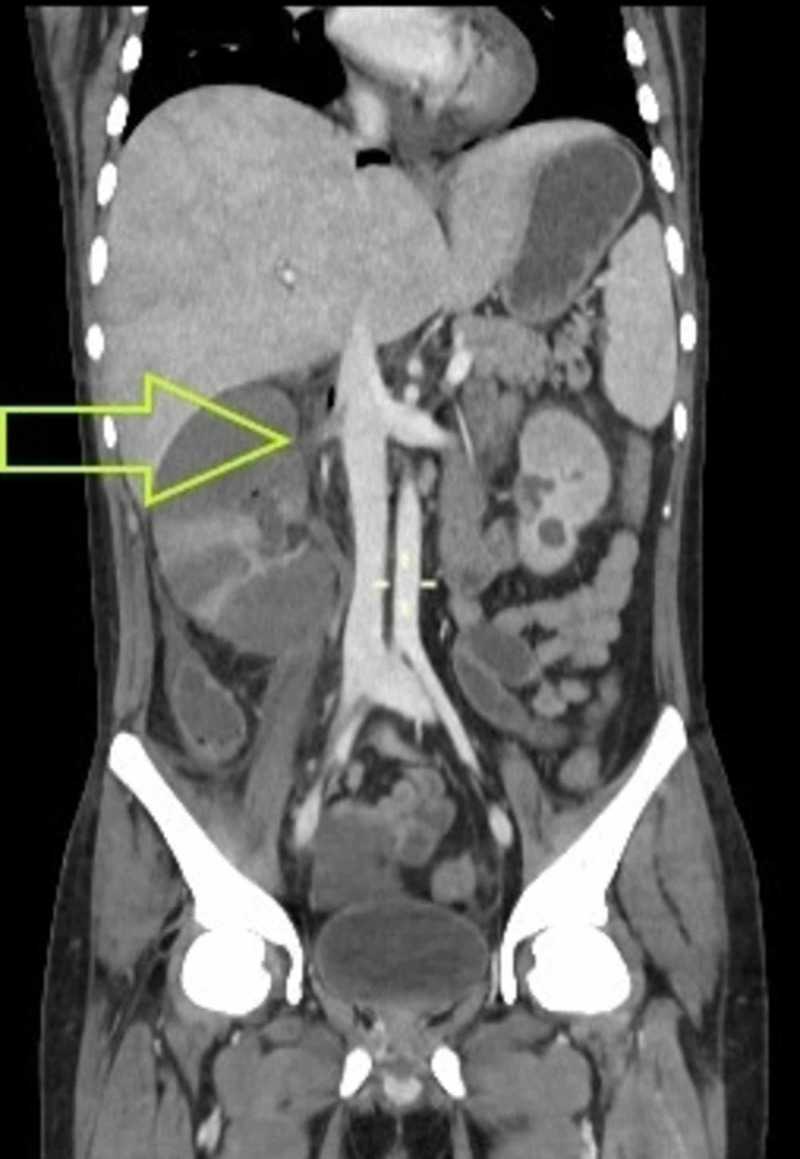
CT with contrast, axial section showing right renal vein thrombosis extending into the inferior vena cava Filling defect is noted involving the right renal vein at it ostium and is seen extending into the hilar region. At the same level, it is seen extending into the inferior vena cava as well. Filling defect is shown by the yellow arrow.
